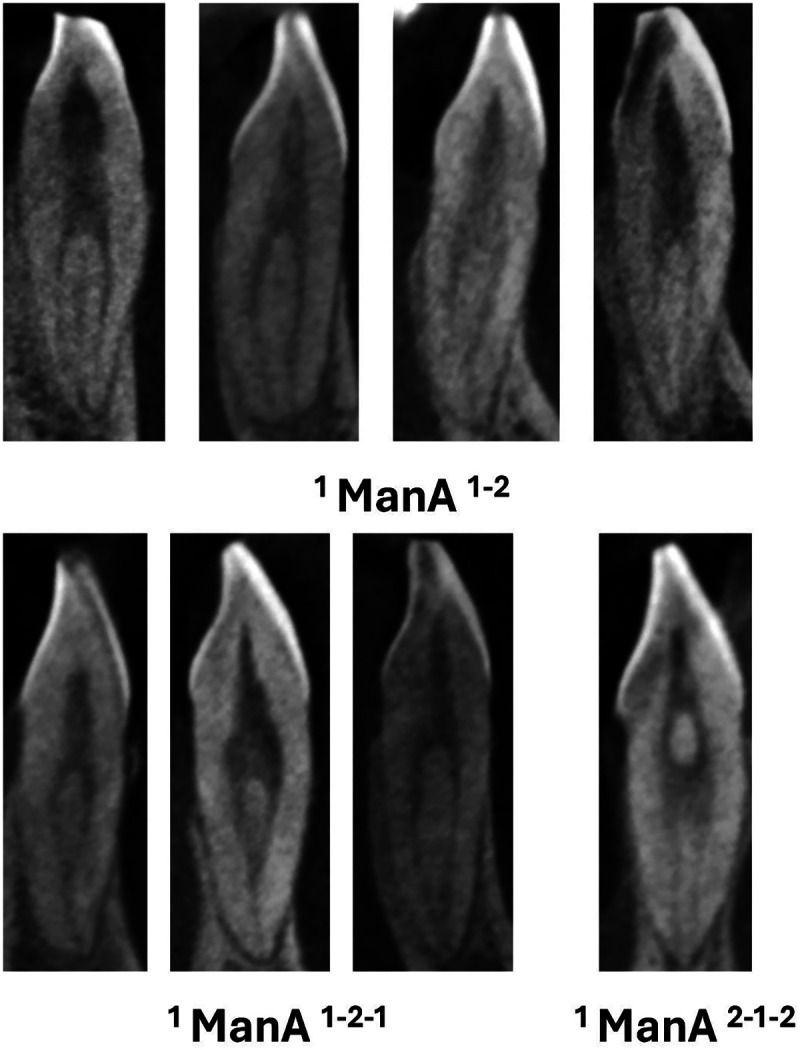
CBCT sagittal view of mandibular anteriors with two and three code classifications (manA-mandibular anteriors).
